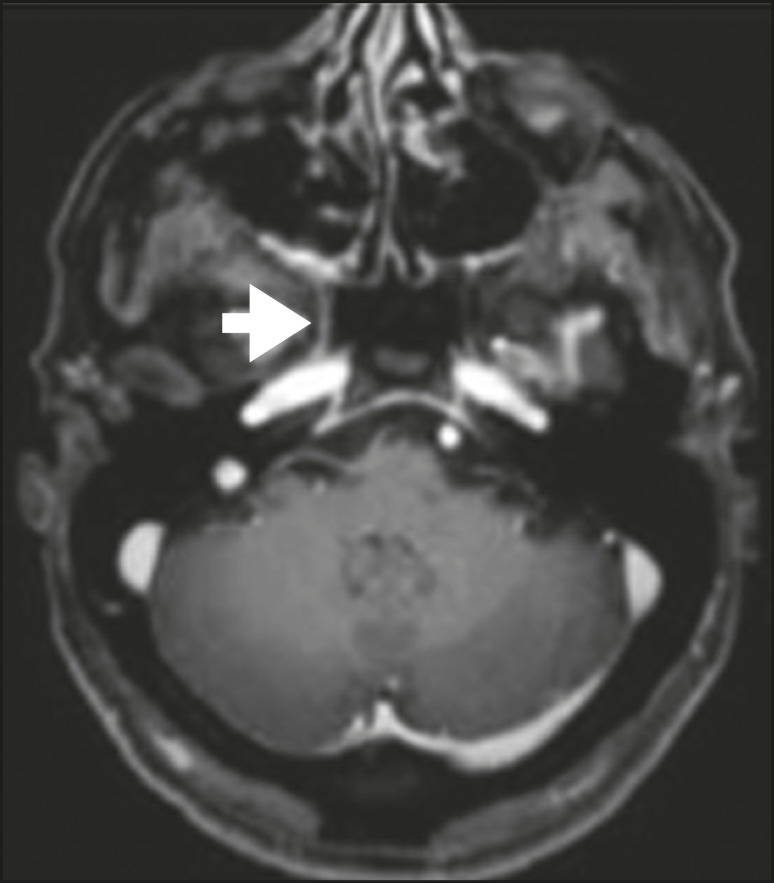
Gadolinium contrast-enhanced T1-weighted MRI scan of a 45-year-old male
